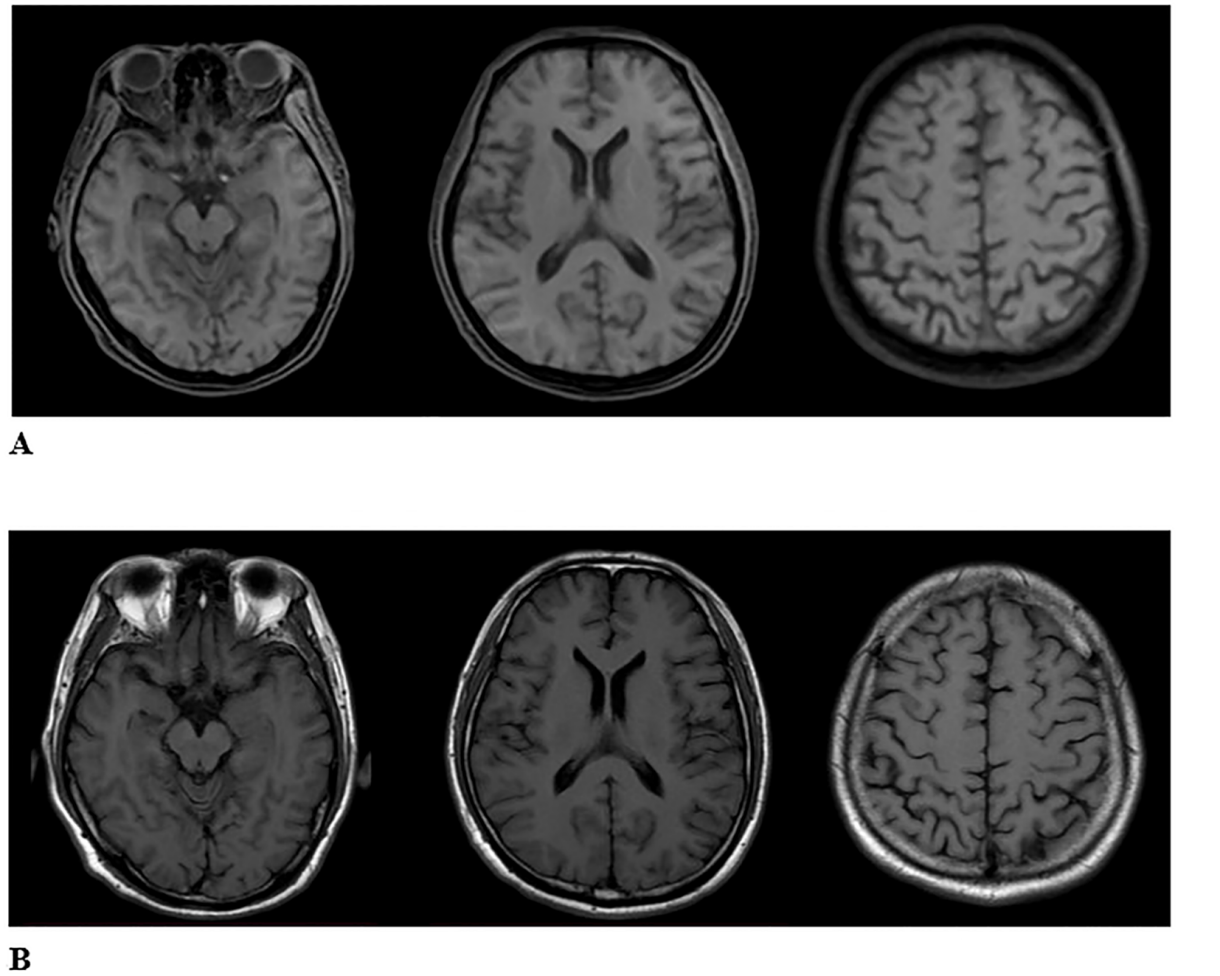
(A) The second image of MRI T1WI in ARFID state showed the bilateral frontal lobes and the right partial parietal lobe were slightly atrophied. (B) The first image of MRI T1WI in healthy state. There is no abnormality.
